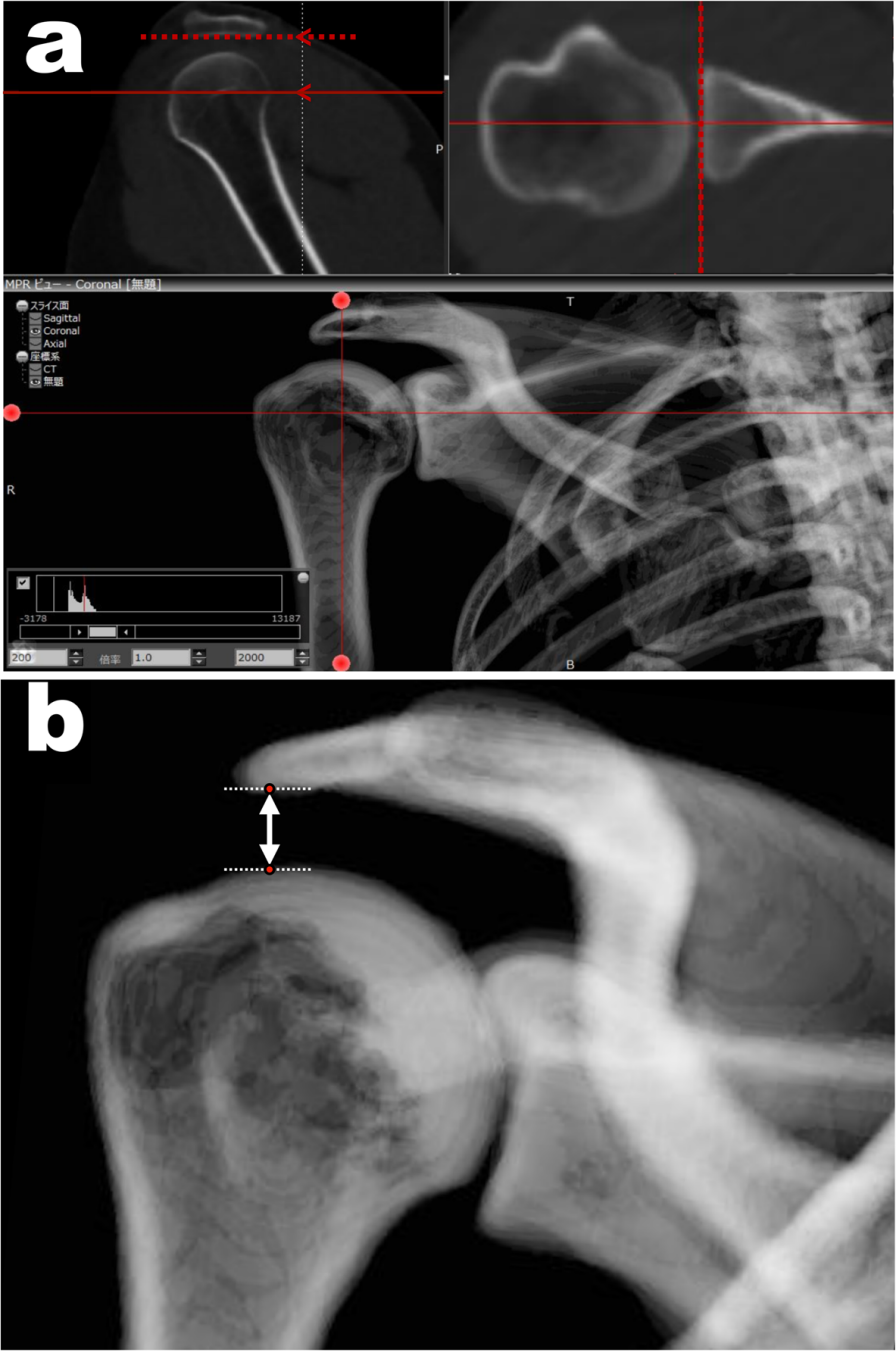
The process of measuring the two-dimensional acromiohumeral distances (2D AHD). a Creating true anteroposterior shoulder digitally reconstructed radiograph (DRR) image from the CT data using ZedView software. Scapula rotation was corrected to align parallel to the inferior aspect of the acromion in sagittal view and align parallel to the face of the glenoid in axial view. b The acromiohumeral distance (AHD) was measured using a two-dimensional (2D) approach on DRR images of the anteroposterior shoulder reconstructed from the CT scans. The 2D AHD was defined as the shortest distance from the dense cortical bone at the inferior aspect of the acromion to the most proximal articular cortex of the humeral head (white line with arrow)
